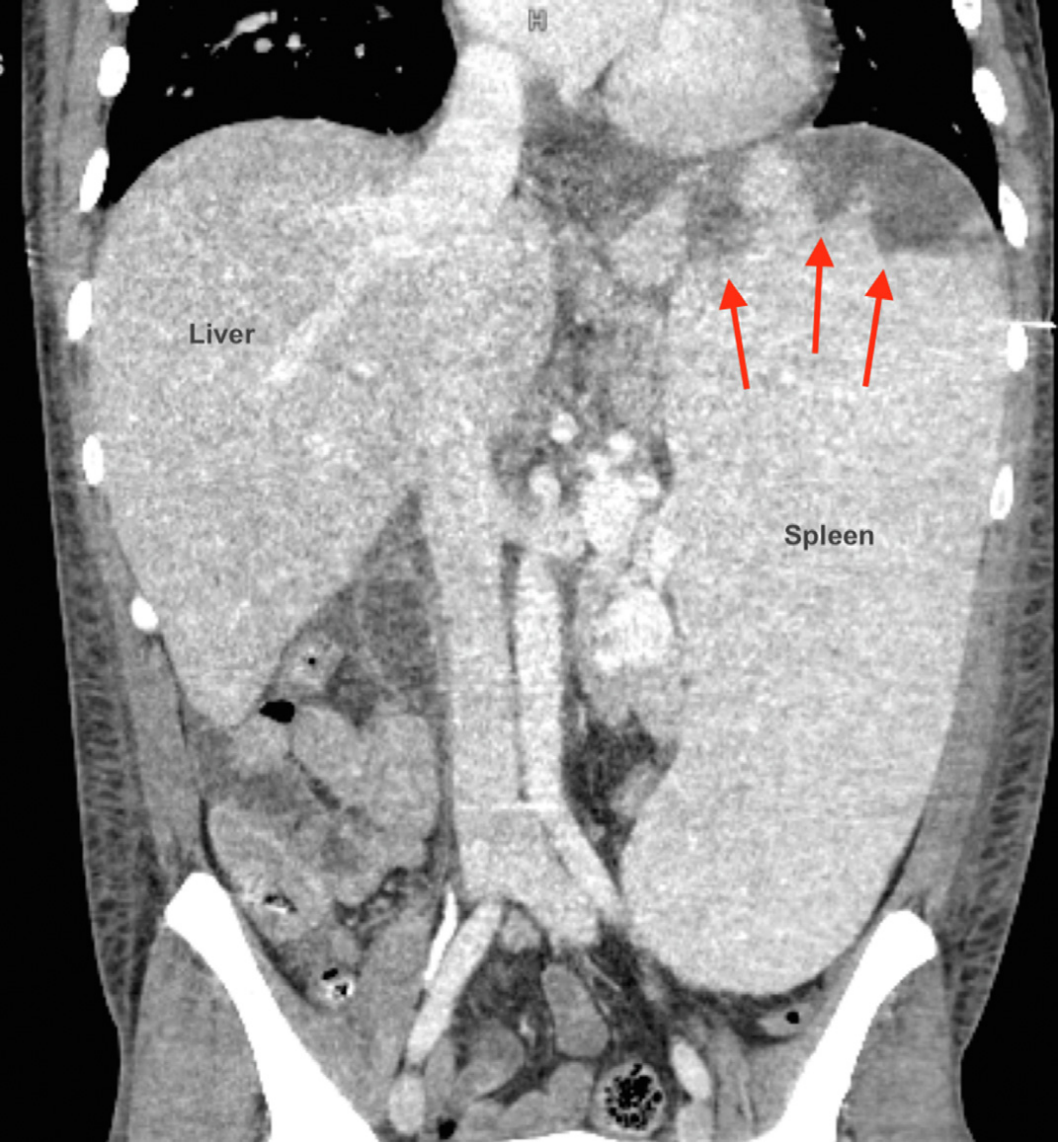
Computed Tomography Angiography of the Abdomen and Pelvis Computed tomography angiography of the abdomen and pelvis on admission showing massive splenomegaly, large wedge-shaped infarcts of the anterior and posterior pole (red arrows), and dilated portal vein suggesting portal hypertension (not pictured).
